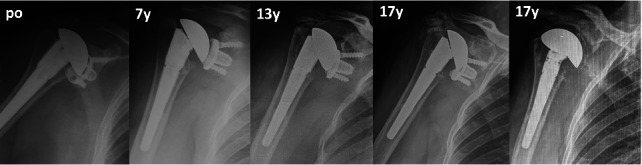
Anteroposterior radiograph views showing a 34-year-old female patient who developed progressive polyethylene eccentric wear with rotator cuff insufficiency noticed at 13 years' follow-up. Conversion to reverse shoulder arthroplasty (RSA) was initially delayed for personal reasons. After 17 years, metal-to-metal contact led to erosion of metal back with severe osteolysis, metallosis with opacification of the periprosthetic soft-tissue (bubble sign^48^ and loosening). Conversion to RSA was not possible, so removal of the baseplate and conversion to hemiarthroplasty was performed. po, postooperative.
